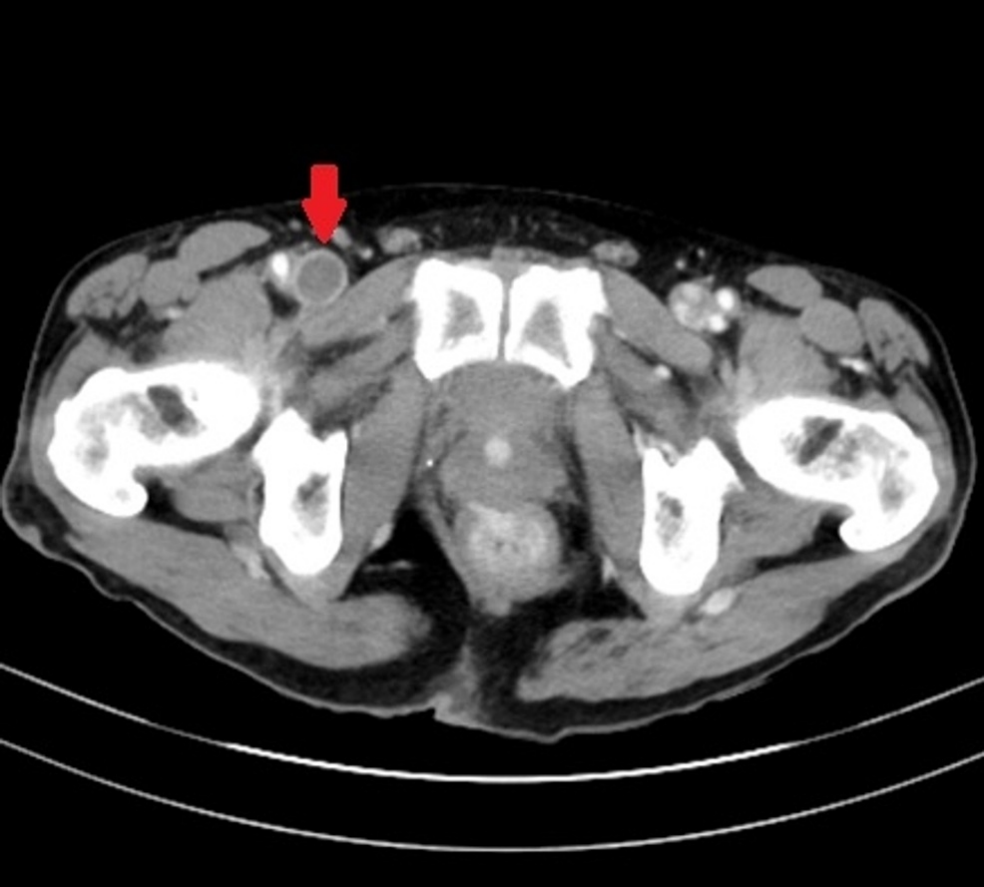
CT of the abdomen and pelvis with contrast in the horizontal plane with the red arrow pointing to deep vein thrombosis of right femoral vein
